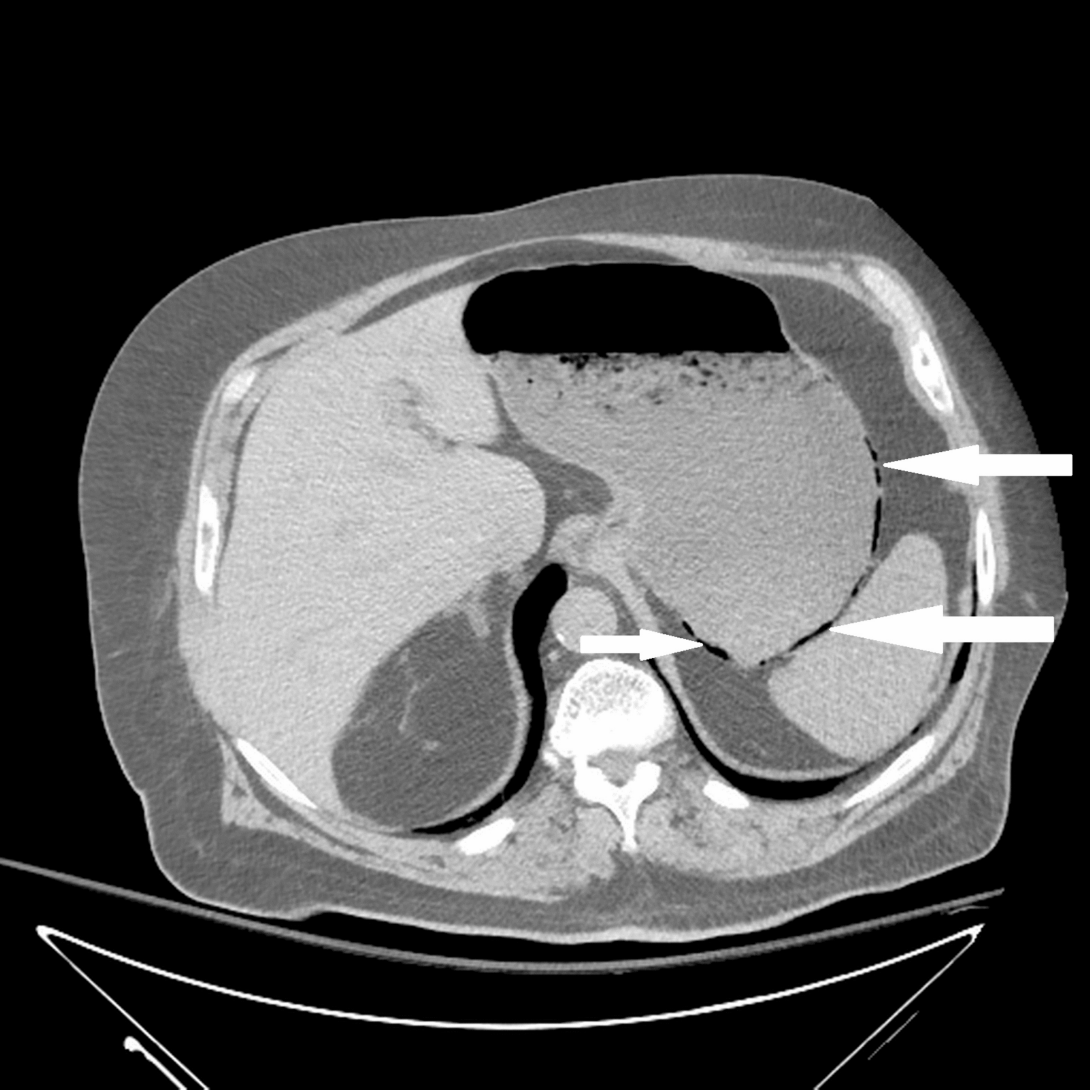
Computed tomography scan, axial view, showing curvilinear air (white arrows) tracking along the posterior wall of the stomach concerning for gastric pneumatosis
